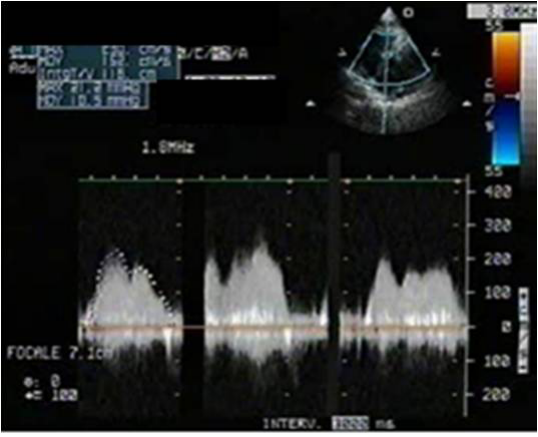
Continuous wave Doppler. The tumor created a hemodynamic tricuspid stenosis which is a sign of high right ventricle inflow velocities.
